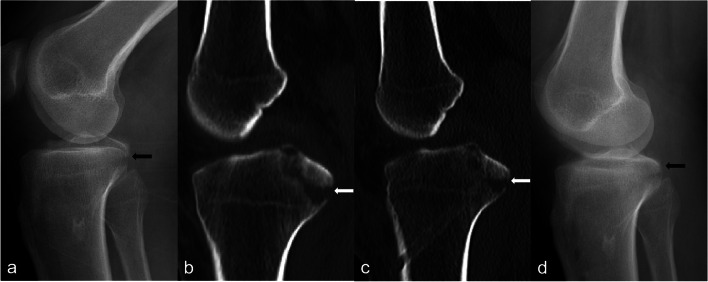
Radiographic examination at preoperative and postoperative. a-b Preoperative X-ray (black arrow) and CT Scans (white arrow) show the elevated bony frament of Type II PCL Tibial Avulsion Fractures. c Immediate postoperative CT scan show the satisfactory reduction of the fracture(white arrow). d Postoperative X-ray at final follow-up showed that reduction of the fracture was satisfactory, and solid union was achieved (black arrow)
